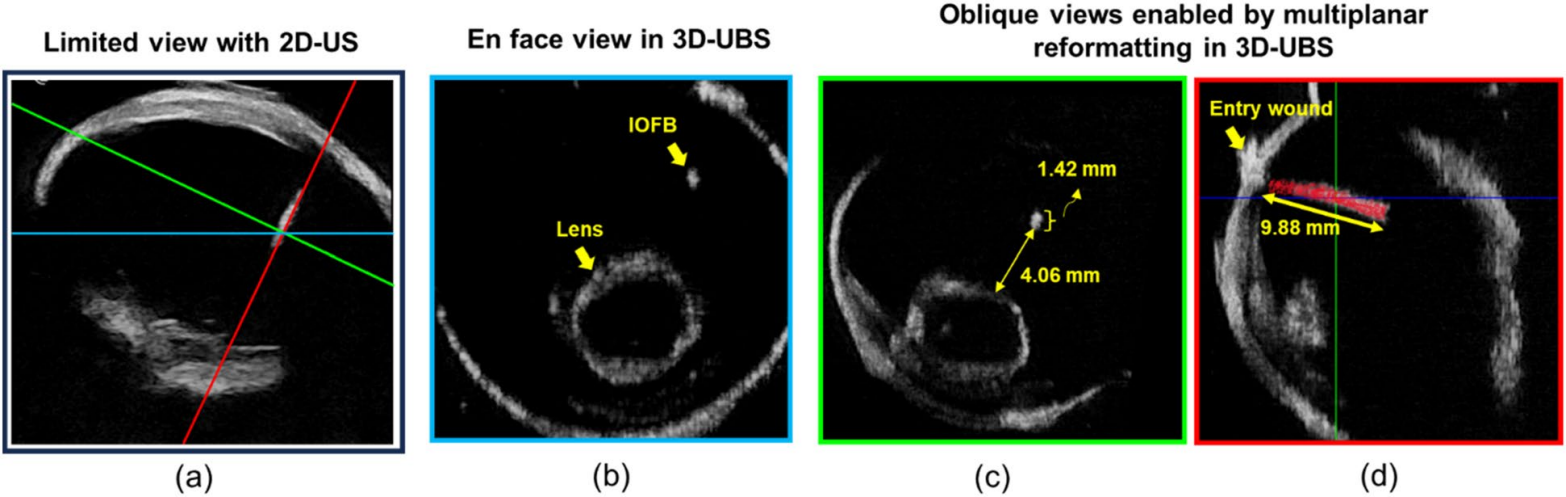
Visualization of a wooden IOFB in 3D-UBS. 2D ultrasound image (a) of the IOFB is limited in information. 3D-UBS allows en face view (blue-b) of the eye, not possible with 2D ultrasound. Multiplanar reformatting of 3D-UBS volume allows visualization of oblique views, (green-c and red-d), that provide more accurate size and location information.
